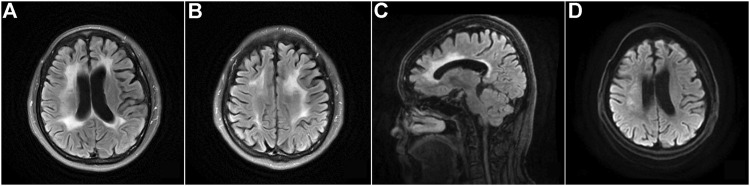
Brain neuroimaging of the proband’s sister (patient Ⅲ-10) with CSF1R-microglial encephalopathy. Bilateral diffuse hyperintense lesions affecting the periventricular white matter, corpus callosum, and deep areas of the frontoparietal lobes on the brain axial FLAIR (A,B) and DWI (D). Sagittal brain FLAIR shows abnormal hyperintensities and thinning of the corpus callosum, and extensive cortical atrophy (C).
